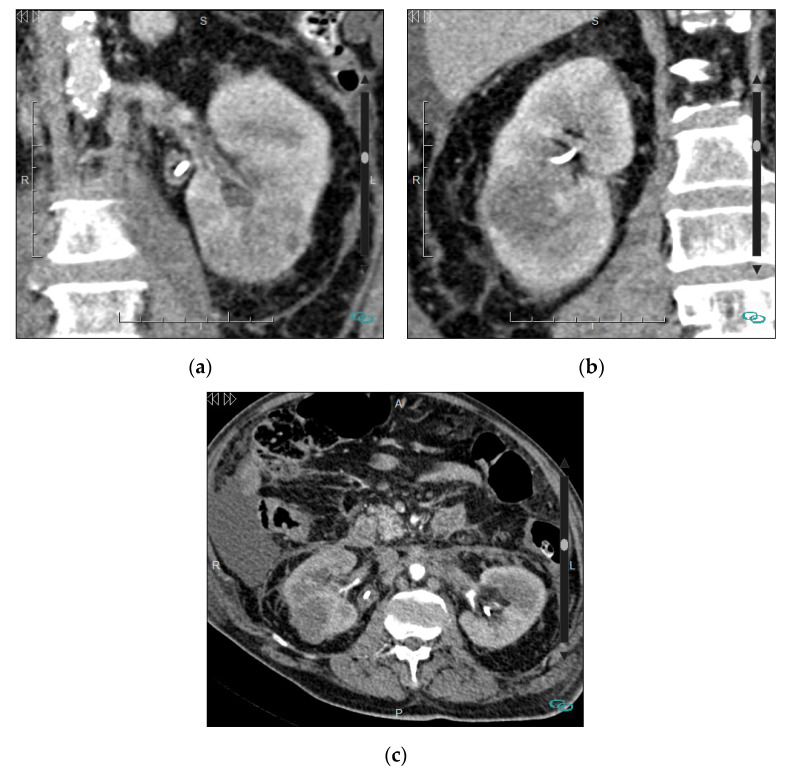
Bilateral metastases in a 65-year-old man. (a) Enhanced coronal CT image in portal phase of the left kidney and (b) right kidney show multiple cortical poorly-defined hypoenhancing lesions; (c) Enhanced axial CT image of the abdomen shows the multiple renal masses and also identifies the presence of stranding and nodularity of the bilateral perirenal fat, thickening of perirenal fascias, multiple retroperitoneal enlarged lymph nodes, and ascites. Diagnosis obtained by biopsy of a renal mass was metastases of high-grade carcinoma of unknown origin. Scale size: 7 cm.
